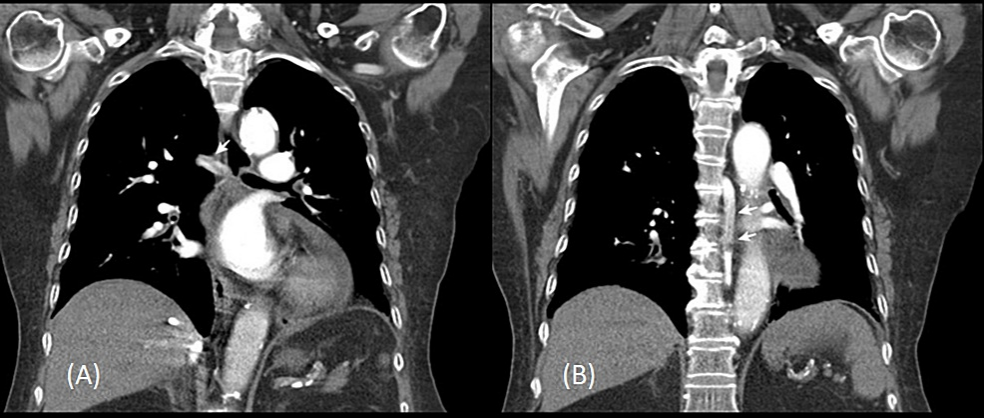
The post-contrast enhanced chest CT in coronal reformation showed partial filling defect in the horizontal (A) and vertical (B) portions of the azygos vein, indicating azygos vein thrombosis (white arrows)
